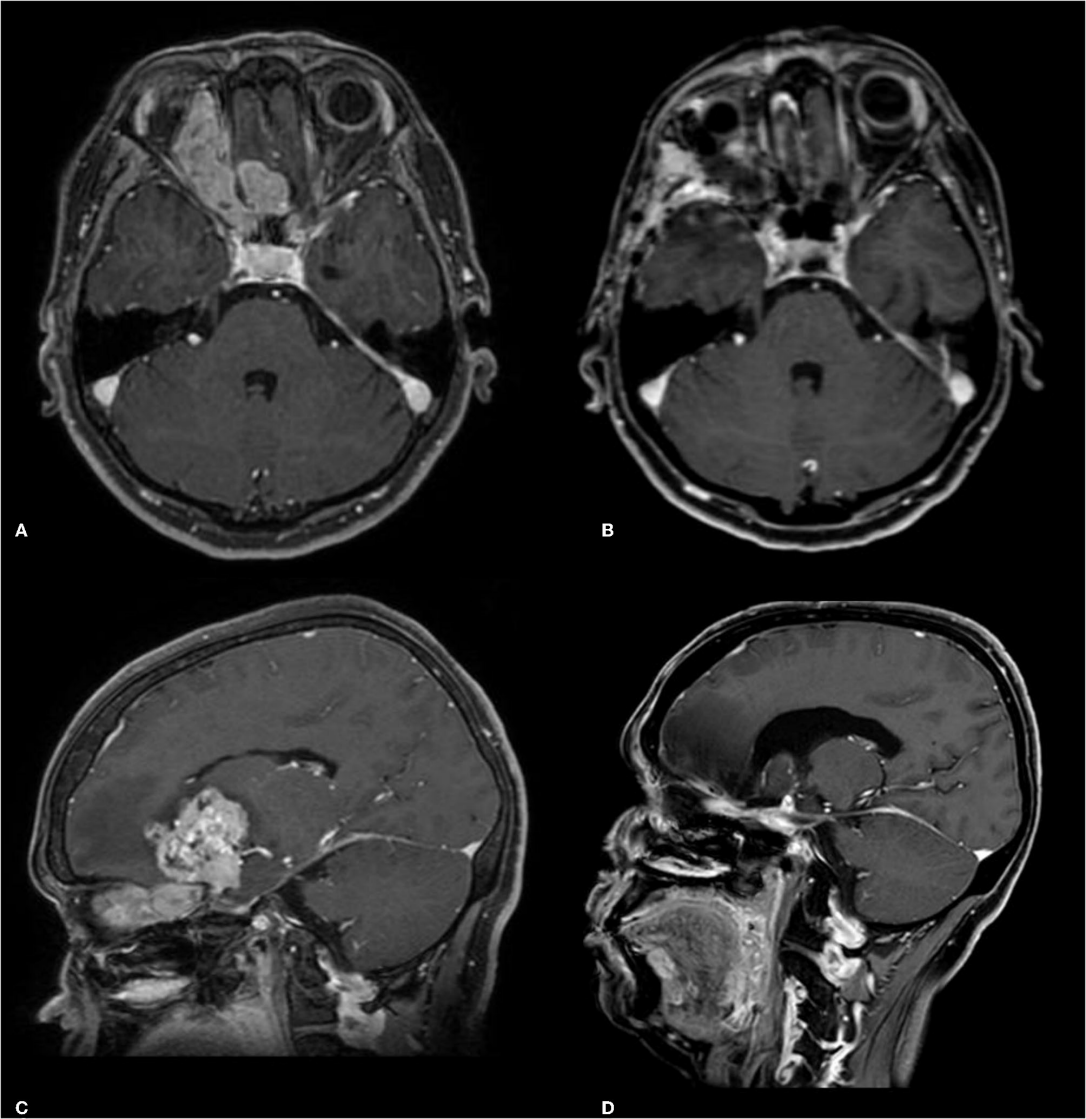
Preoperative post-contrast T1-weighted MRI (A,C) and postoperative images (B,D).
